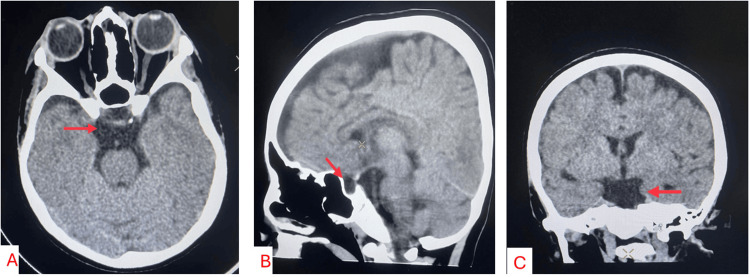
a, b, c) Axial, sagittal, and coronal views: CT scan performed 10 days after surgery; arrow demonstrates radical tumor removal
